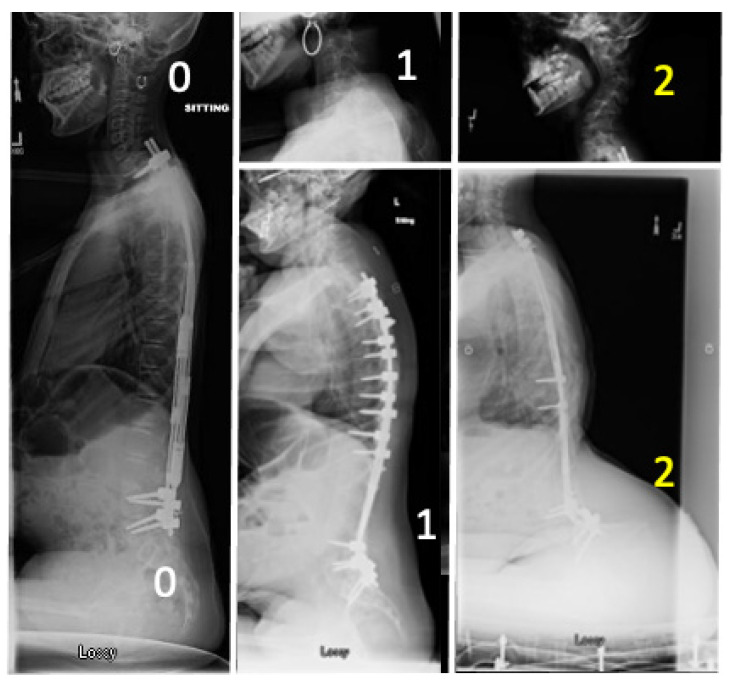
Examples of scoring the latest available radiographs in this study. (Left) Normal (0,0) scoring of no tucked chin or trunk tip. (Middle) Transitional scores of 1 for slight visual tucked chin (top) and prominent buttock (bottom). (Right) Obvious tucked chin (top) and tipped trunk (bottom) scoring a 2 in our system. Only those scoring a 2 (denoted in yellow) were included in our Deformity Cohort, these were compared against those scoring 0 or 1 (white).
